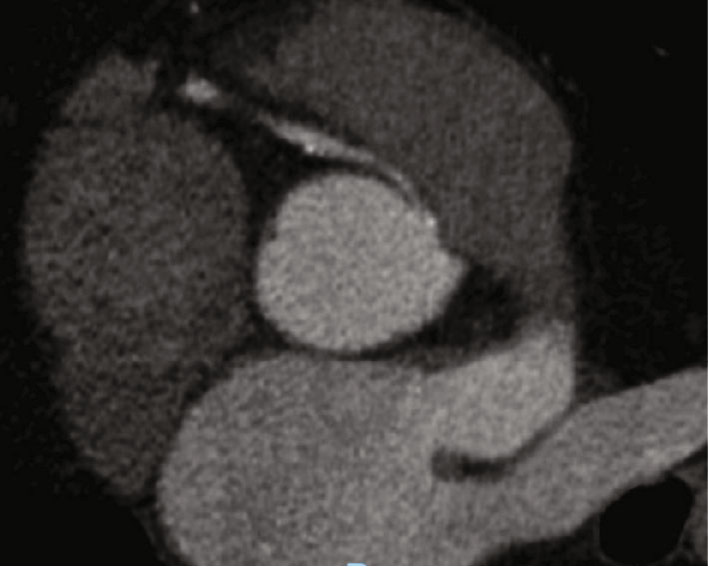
CT angiogram reveals the anomalous origin of the right coronary artery of the left coronary cusp with a slit-like opening and an interarterial course.
